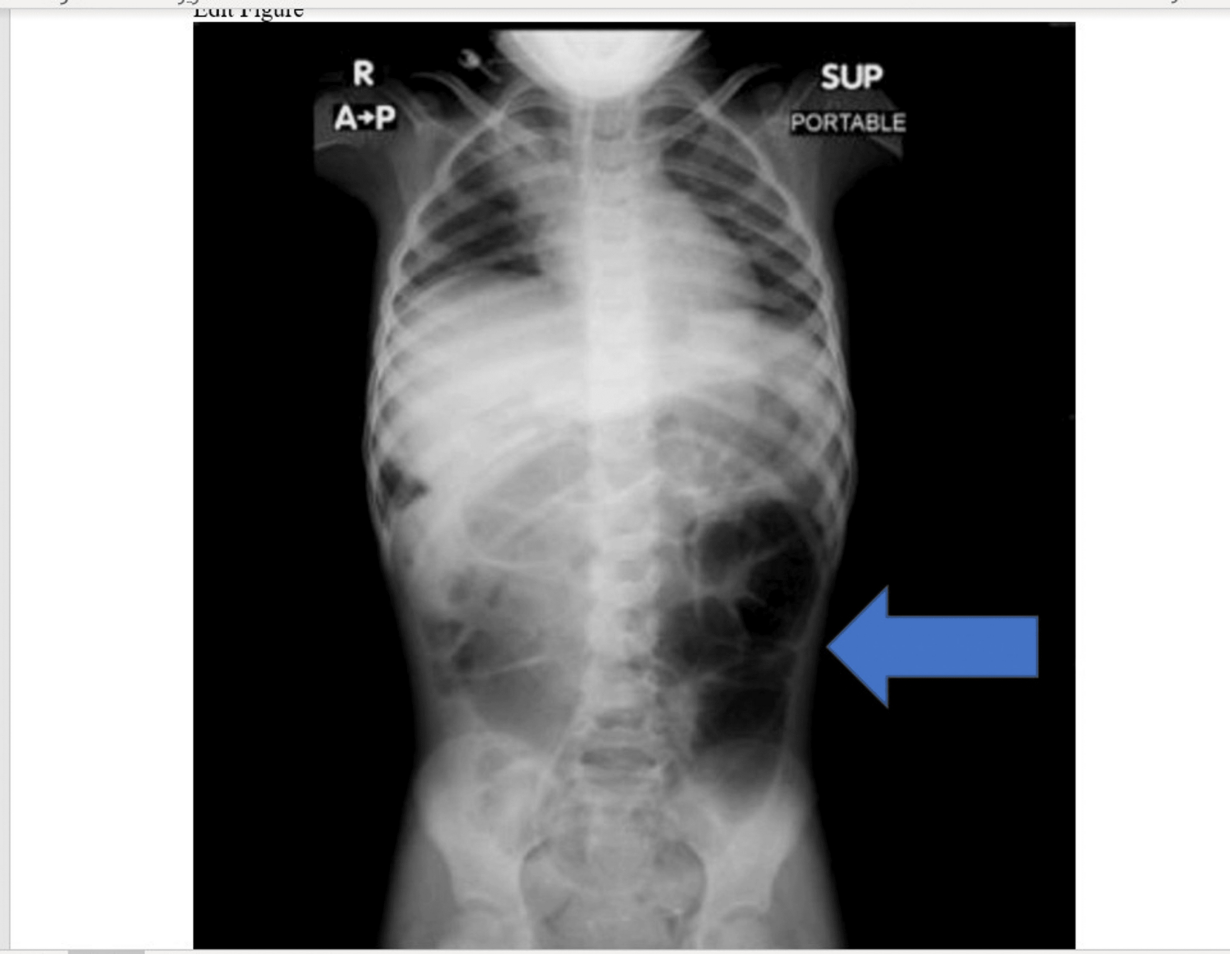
Abdominal X-ray shows moderate dilatation of bowels Antero-posterior erect abdominal X-ray showing moderate dilatation of bowels as shown by the arrow.
